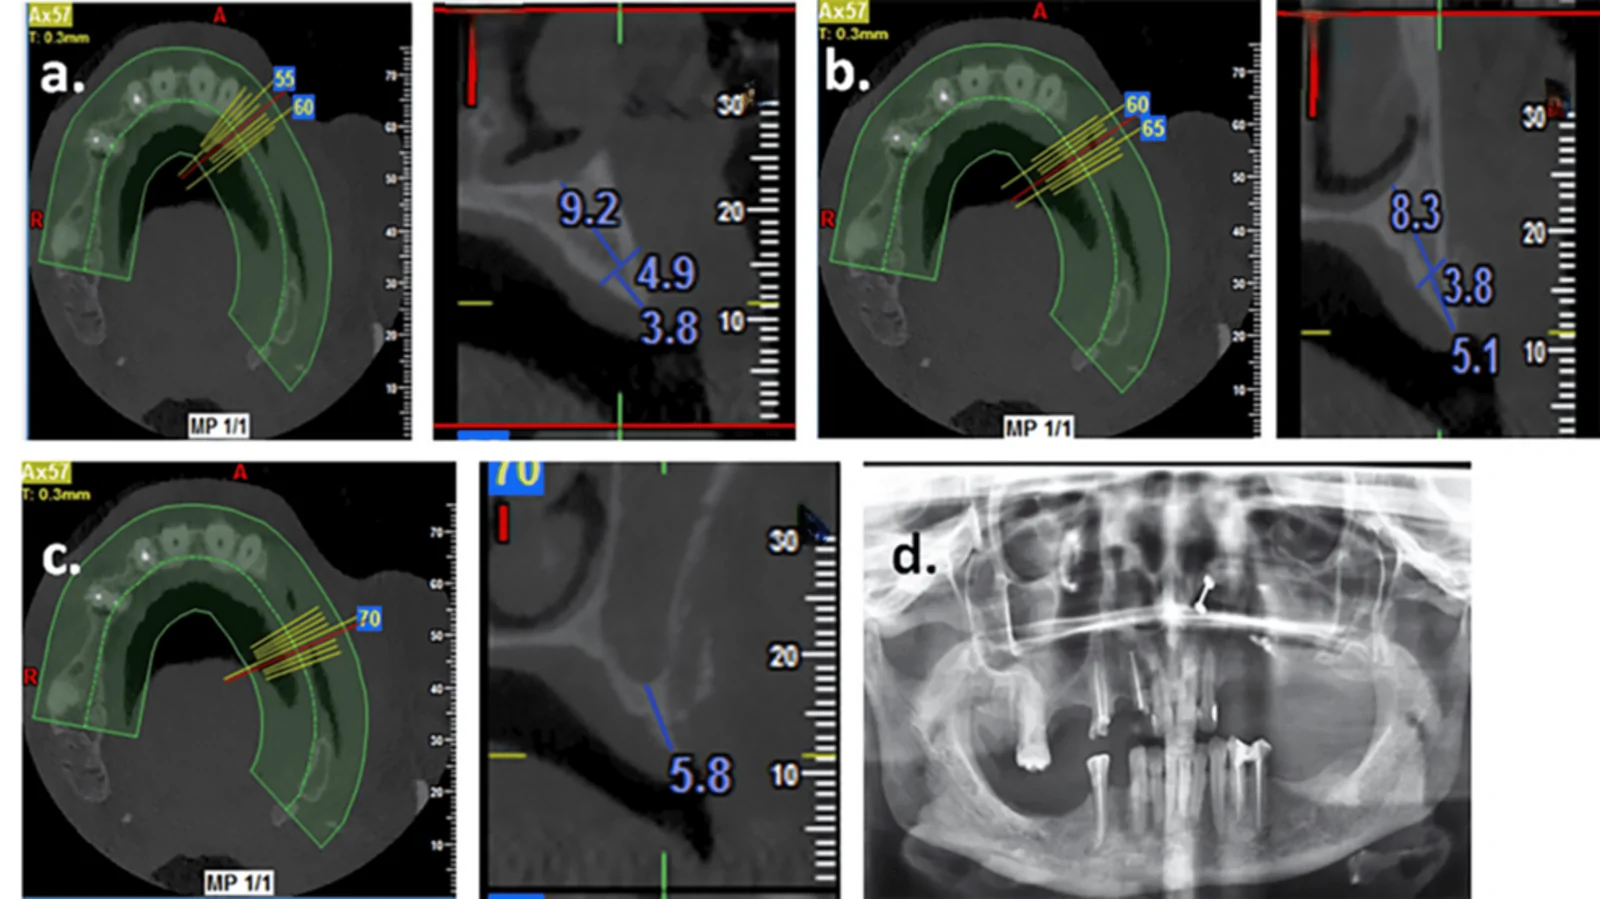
Postoperative assessment for implant placement at the implant sites. a) CBCT of region 23. (b) CBCT of region 25. (c) CBCT of region 27. (d) OPG X-ray image after sinus augmentation. CBCT: cone beam computed tomography; OPG: orthopantomogram
